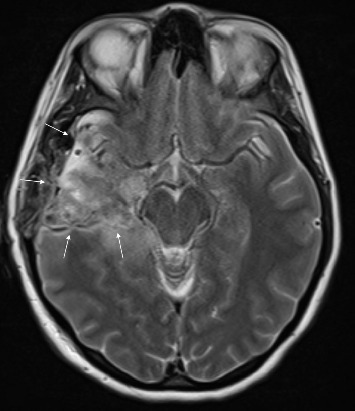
Postoperative day one MRI T2-weighted sequence, demonstrating extensive surgical resection (denoted by arrows) with a small T2 abnormality in the right hippocampus and surgical hemostatic product and a small amount of blood within the resection cavity.
